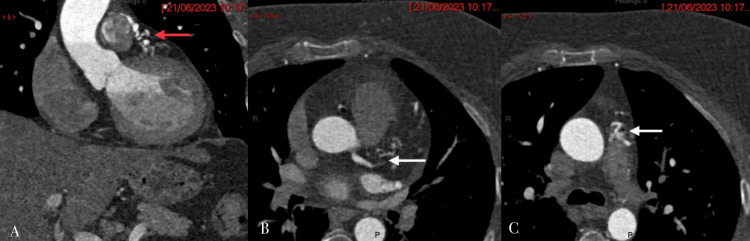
(A) Coronal and (B, C) axial CT images show multiple dilated, tortuous fistulous tracts (red arrow in A) arising from the left anterior descending artery (arrow in B) and the right conal artery, draining into the main pulmonary artery (arrow in C).
